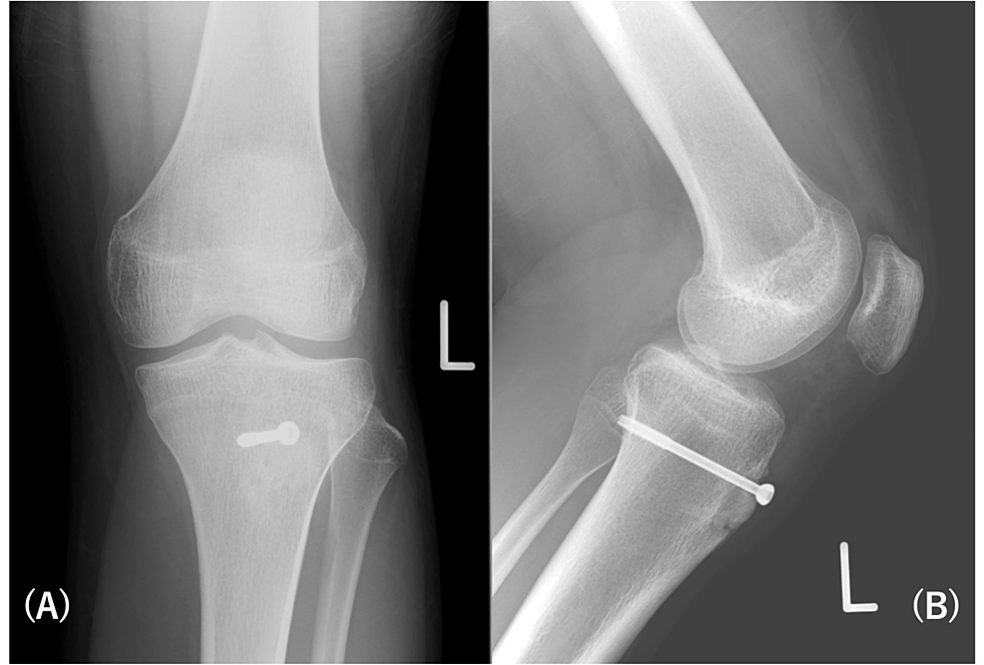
Six months postoperative radiograph.
